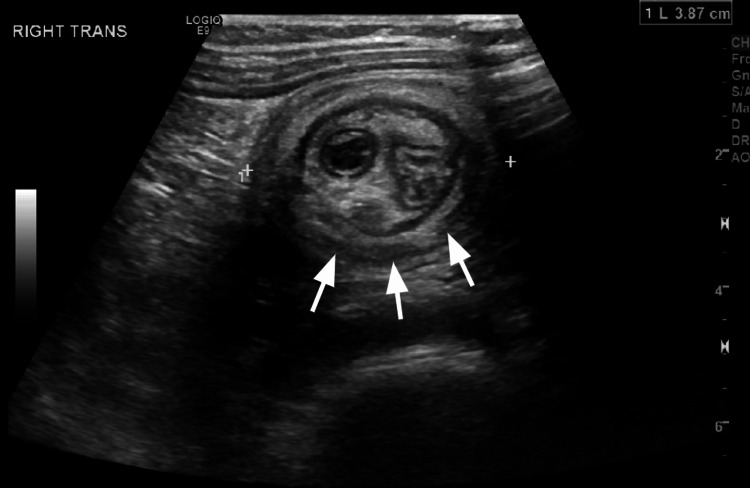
Abdominal ultrasound image The image shows ileocolic intussusception with a dilated appendix measuring 8 mm in diameter.
